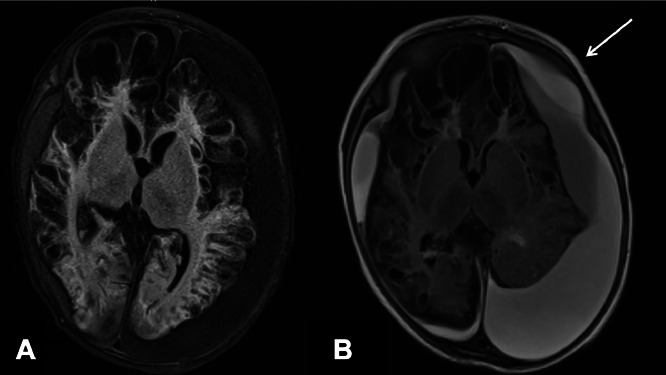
(A) Repeat axial FLAIR MRI of the brain without contrast at 22 days showing diffuse frontoparietotemporal predominant encephalomalacia. (B) Repeat axial FLAIR MRI of the brain without contrast at 2 months after presentation with diffuse severe cystic encephalomalacia of the entire brain with white arrow indicating more severe encephalomalacia in the left hemisphere than the right with associated subdural fluid collections.
